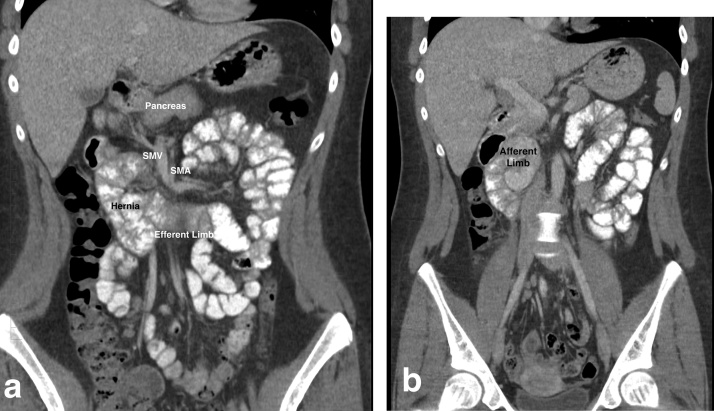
CT Images of right paraduodenal hernia. (a); Right paraduodenal hernia showing sac like mass of small bowel loops to right of midline and efferent limb crossing back over to left side of abdomen. (b); Afferent limb of proximal jejunum in upper right abdomen. SMV, superior mesenteric vein; SMA, superior mesenteric vein.
